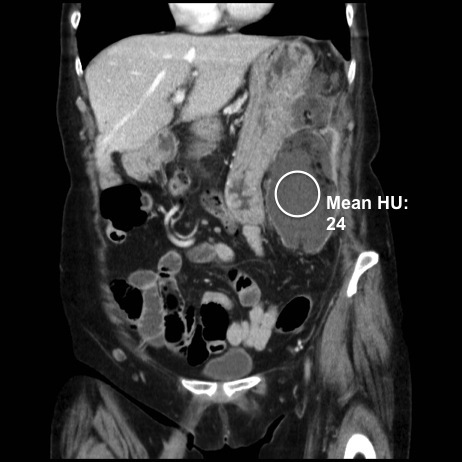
Coronal portal venous phase contrast-enhanced CT: circle represents region of interest measurement tool within left upper quadrant liquefied collection with foci of gas.
